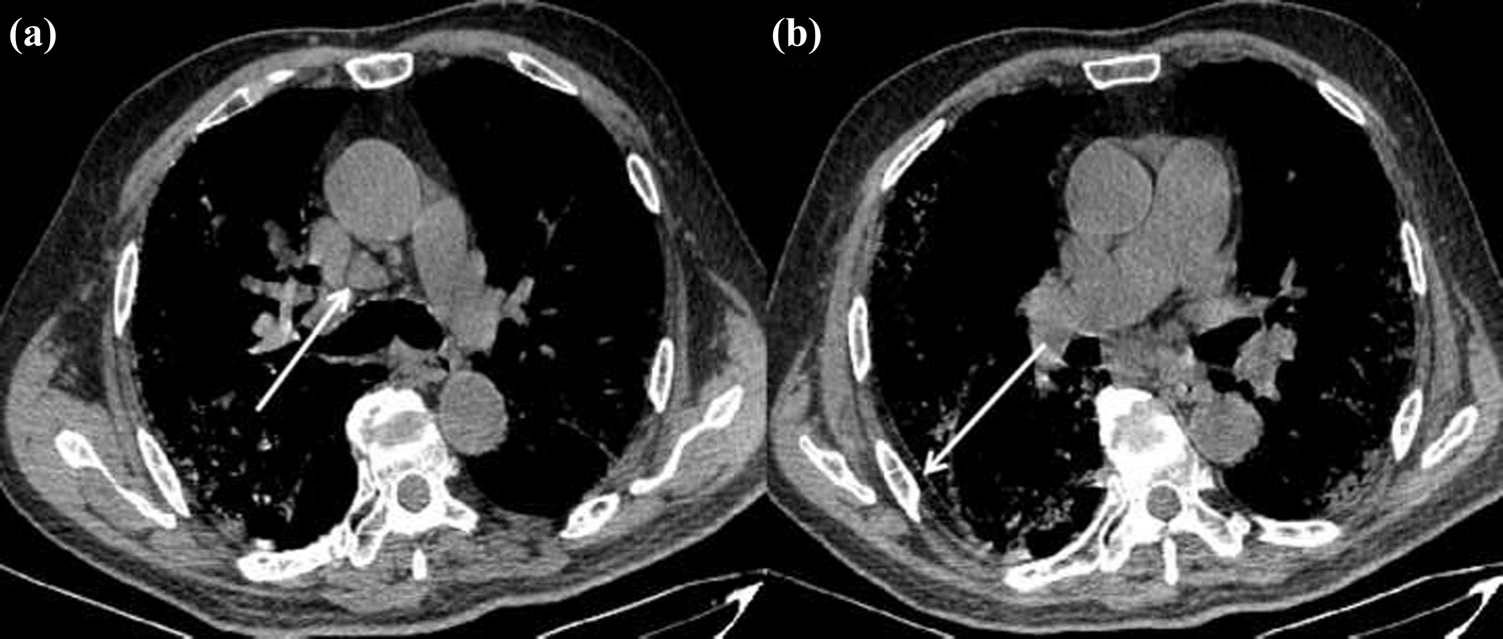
First CT scans (axial mediastinal noncontrast image) showing mediastinal enlarged lymph nodes (white arrow, a) and local thickening of the pleural (white arrow, b).
